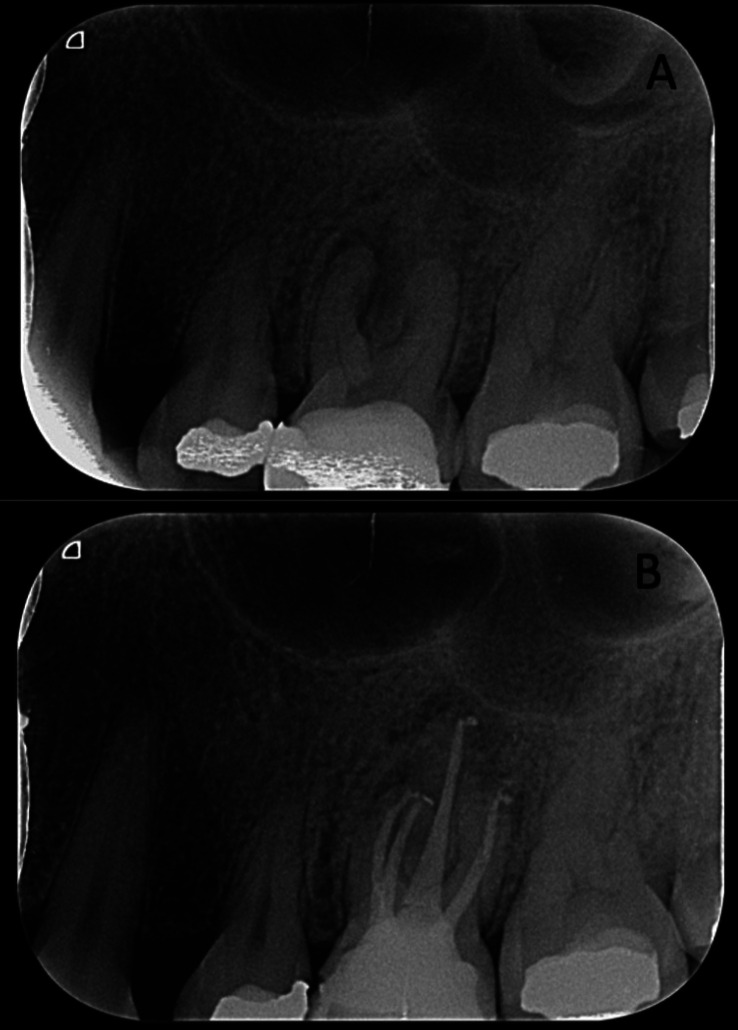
A) Initial and B) final radiographs of the root canal treatment in the maxillary left first molar with location of the MB2 root canal.
